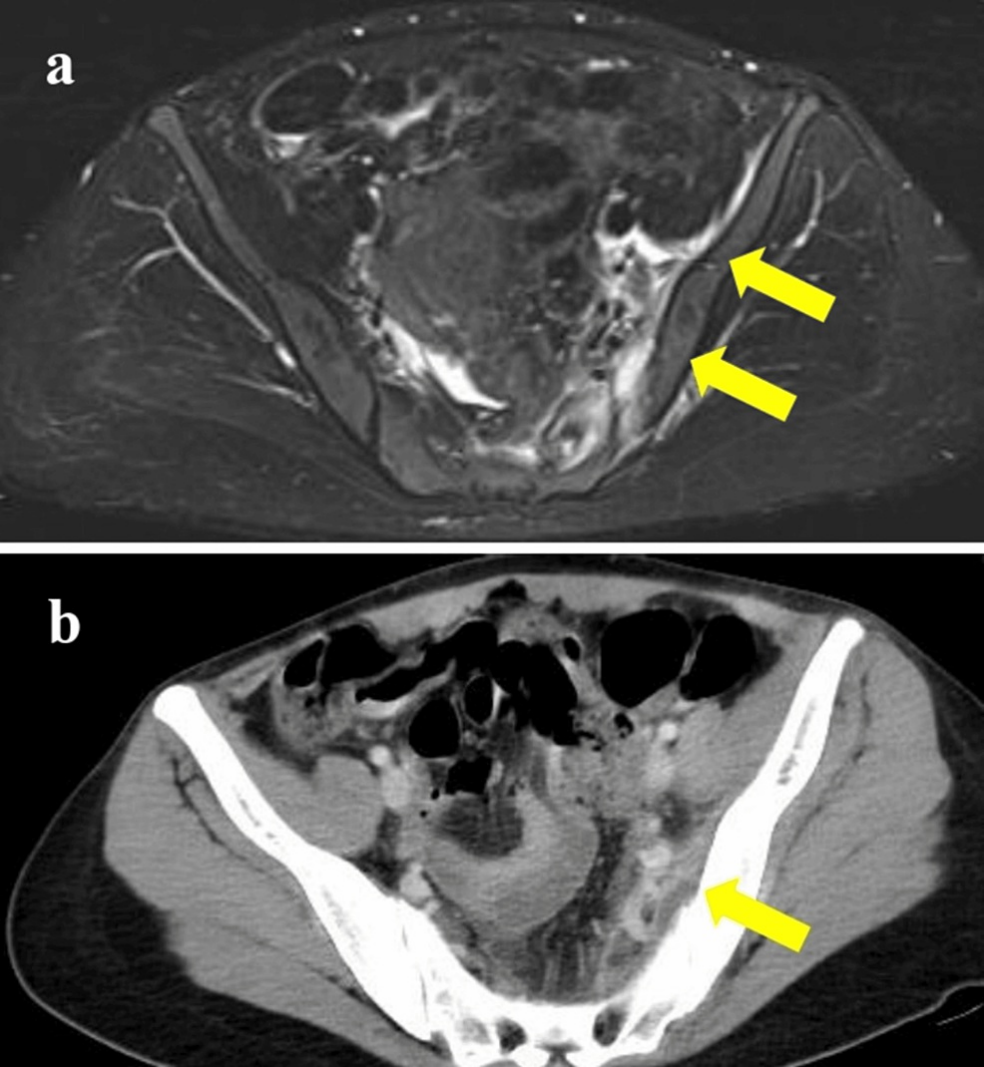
(A) Magnetic resonance imaging of the hip joint and (B) computed tomography of the pelvis. (A) Inflammation in the left iliacus muscle was demonstrated by an MRI (short tau inversion recovery) taken on day three of admission. (B) Abscess and fluid collection adjacent to the left ileum were demonstrated by CT taken on day four of admission.
